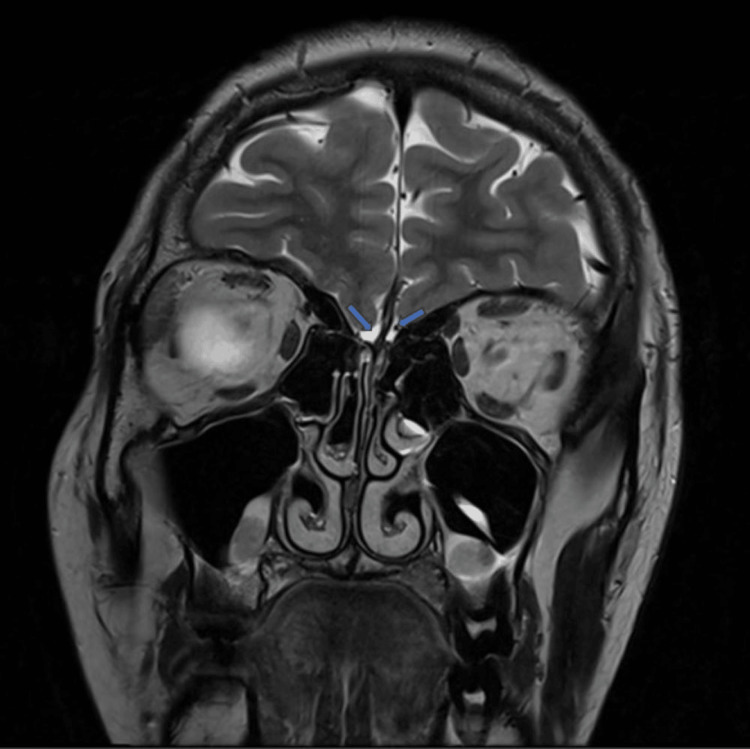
Coronal T2-weighted MRI shows absent olfactory bulbs in bilateral olfactory grooves with hypoplastic olfactory sulci on both sides (blue arrows).
